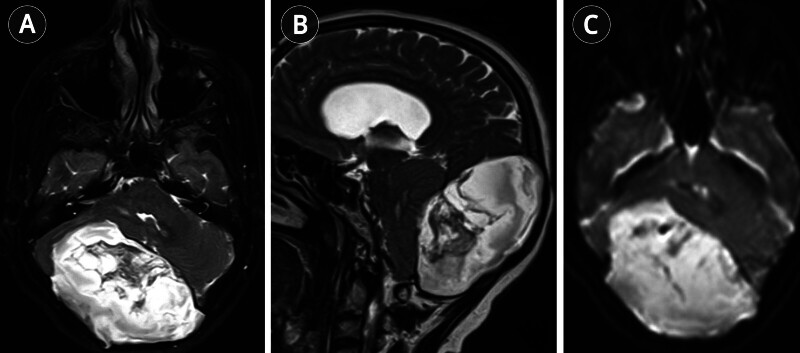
Preoperative MRI of the brain. (
